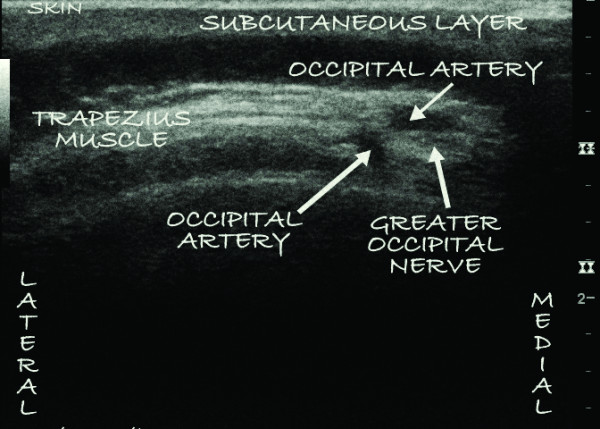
An ultrasound image of the left occipital nerve as well as two divisions of the greater occipital artery as they pierce the substance of the trapezious muscle 1.0 cm to 1.2 cm from the skin.
